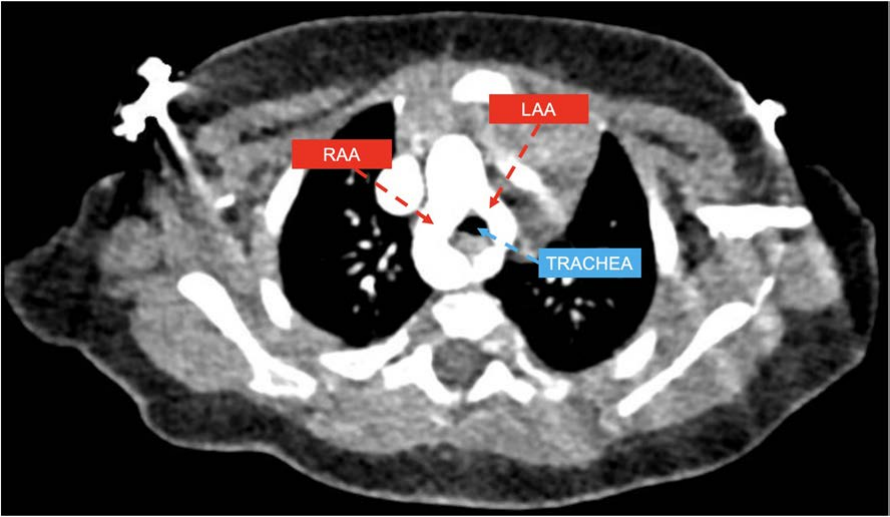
CT axial view showing double aortic arch with dominant right aortic arch (RAA) and hypoplastic left aortic arch (LLA) compressing the trachea.
